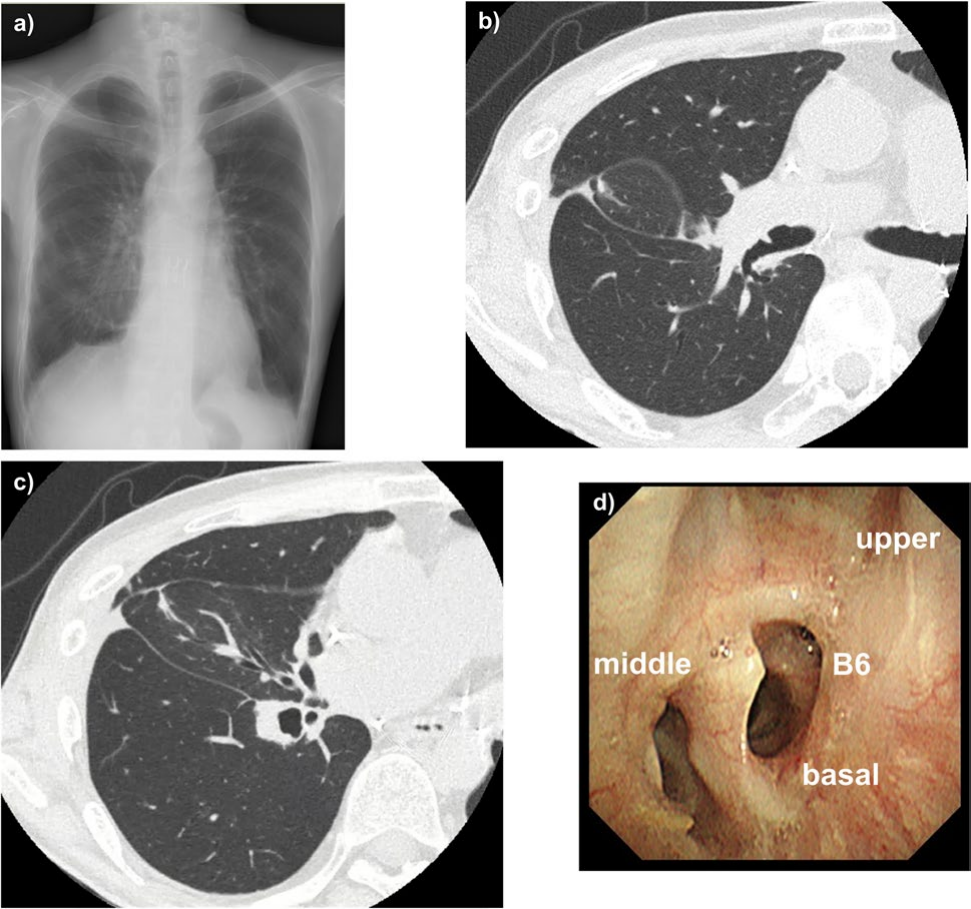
Postoperative radiological and bronchoscopic findings at 2 months after surgery. a Chest radiography showing improved expansion and aeration of the right lung. b, c Chest computed tomography images demonstrating well-aerated right middle and lower lobes with patent bronchi extending to the periphery. d Bronchoscopic findings showing good patency of the middle and lower lobe bronchi with no mucosal edema or restenosis
